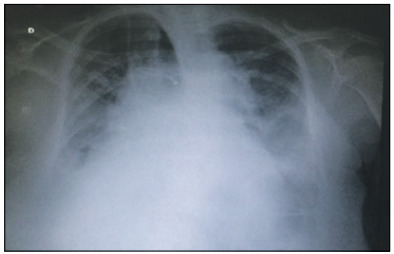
Chest X-ray in posterior-anterior view (April 24, 2015).
